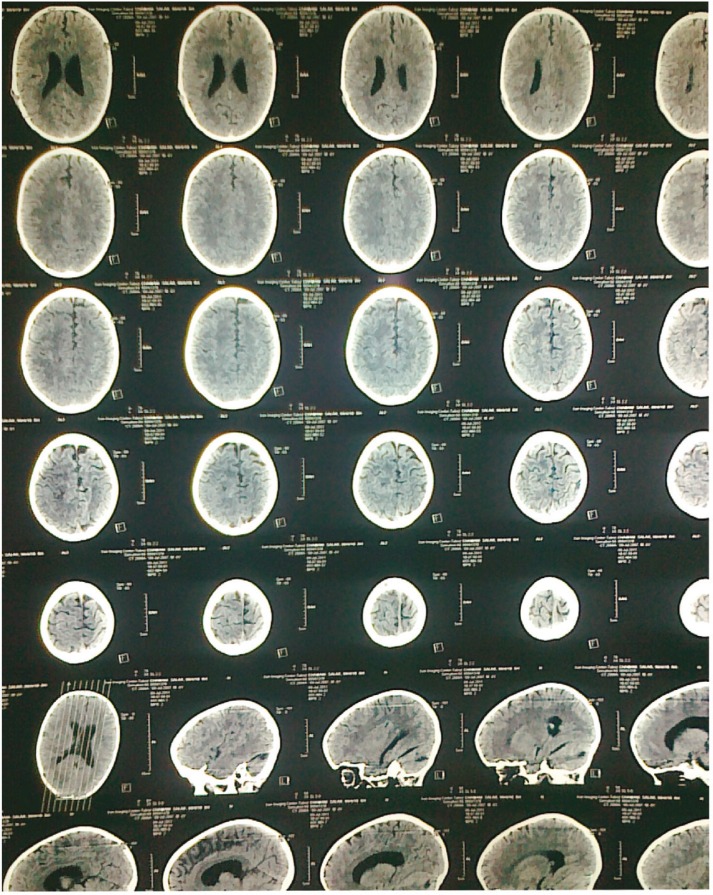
Brain CT scan after extraction of ventriculoperitoneal shunt, there was no changes in hydrocephalus
